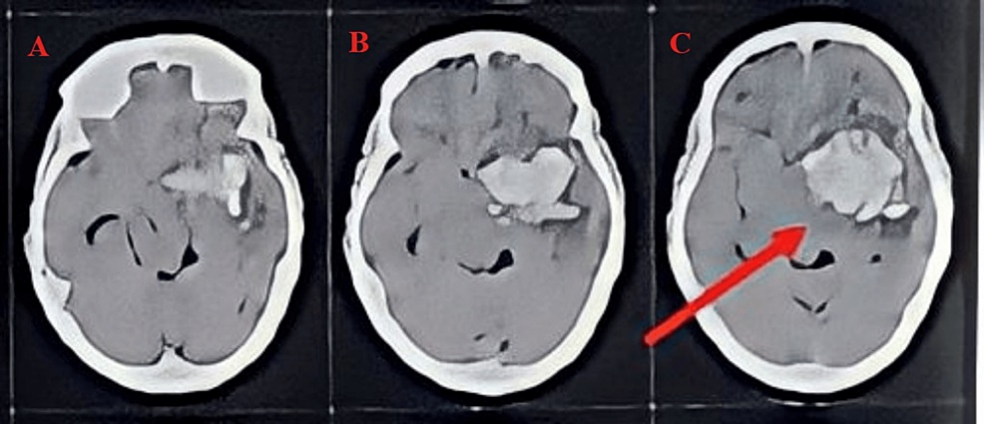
Computed tomography scan showing intracranial bleed. (A) Bony defects on the right frontal, parietal, and temporal regions. (B) Hemorrhage in the gangliocapsular region. (C) Midline shift of 9 mm.
